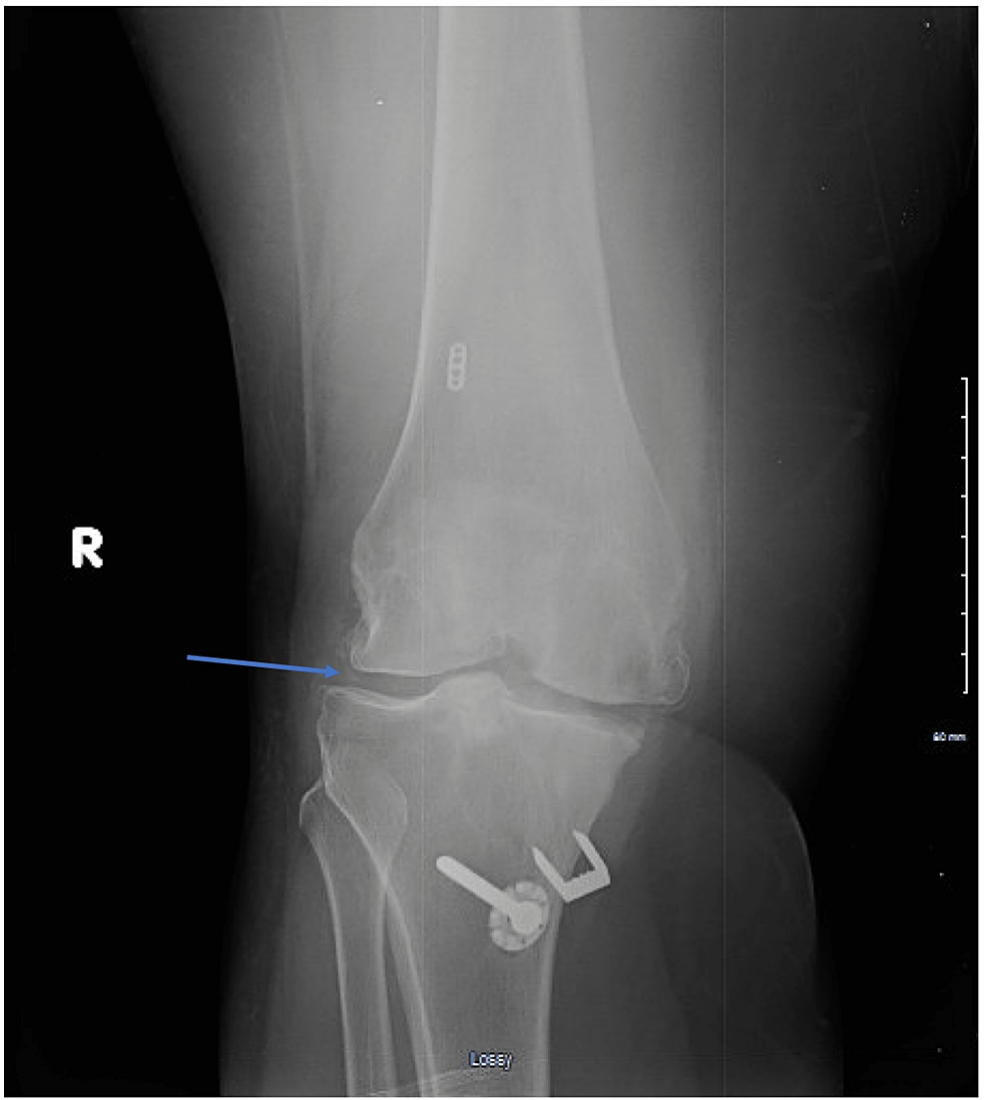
Plain radiograph of the right knee demonstrating mild meniscal chondrocalcinosis due to calcium pyrophosphate dihydrate deposition.
